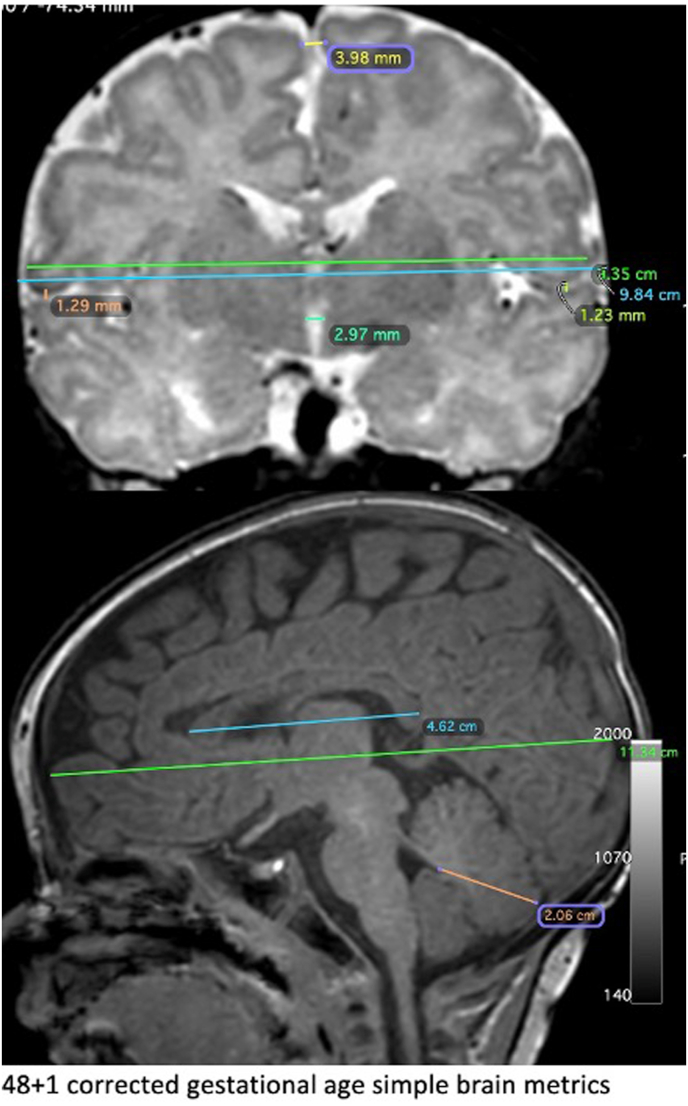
Example of a neonatal MRI with simple brain metrics measured.
